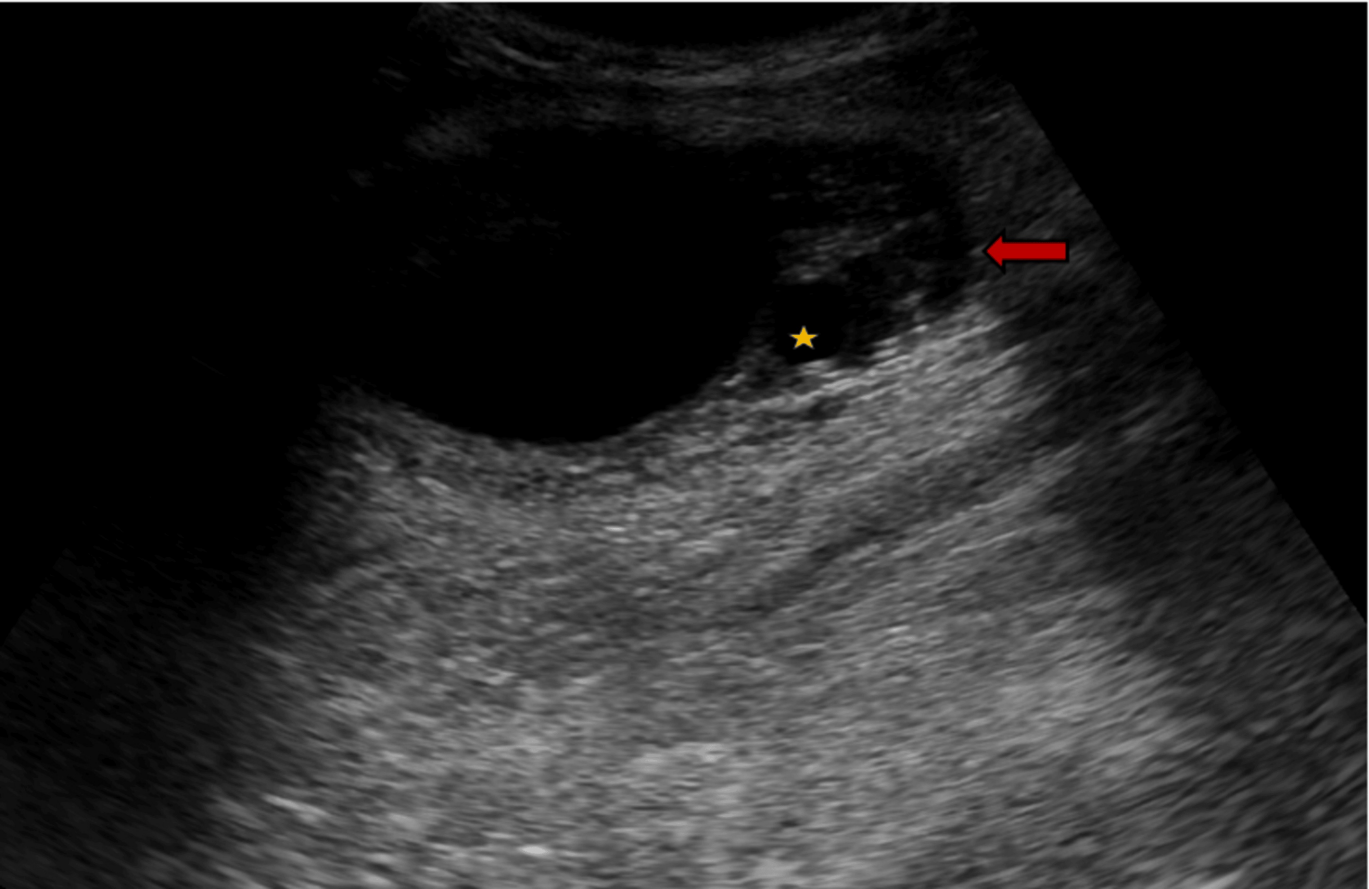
Ultrasound image showing well-defined oval cystic lesion (arrow) with multiple small cystic lesions (star) adjacent to it within the muscle.
